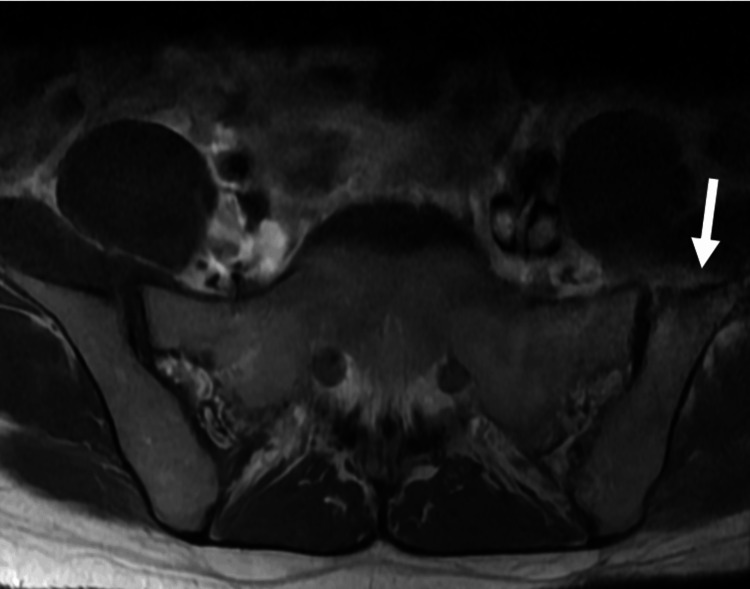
Transverse lumbar spine MRI with contrast, demonstrating a mild T1 hypointense marrow signal along the periphery of the left SI joint with possible joint erosion (white arrow) MRI: magnetic resonance imaging; SI: sacroiliac
